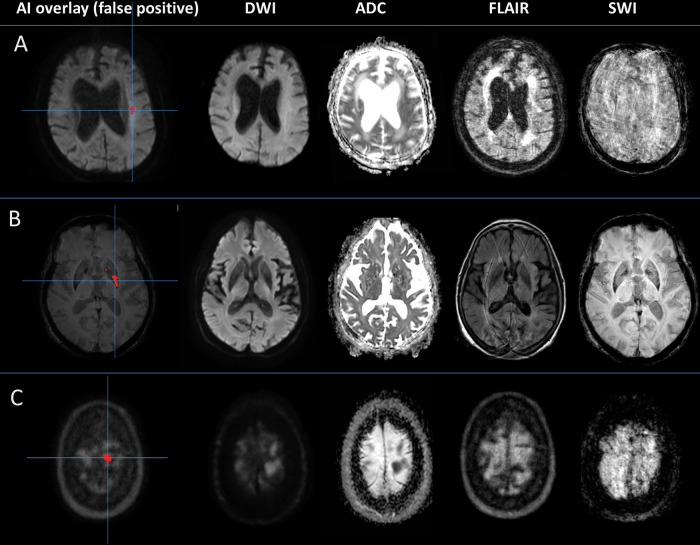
False positive predictions from AI tool in scans with motion artifacts. The left column displays the AI interface with the overlay and lesion mark. The right columns display sequences from PACS (DWI, ADC, FLAIR, and SWI). A 77-year-old female with decreased consciousness. Stroke MRI shows no ischemic lesions, while AI-overlay shows a false positive ischemic lesion. B 83-year-old female with impaired left-sided vision. Stroke MRI shows no hemorrhagic lesions, but AI-overlay shows false positive hemorrhagic lesions. C 74-year-old female with dysarthria and facial paresis. Stroke MRI was without space-occupying lesions, but AI-overlays show a false-positive space-occupying lesion
